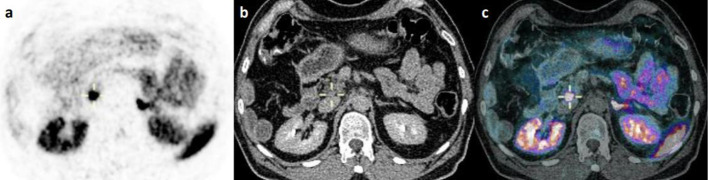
^68^Ga-DOTATATE PET/CT images showing extrinsic compression of the uncinate process of the pancreas by a pre-cave adenopathy in a 55-year man with grade I midgut NET with metastatic spread; a Axial PET slice, b Axial CT slice and c Fused axial PET/CT slice
